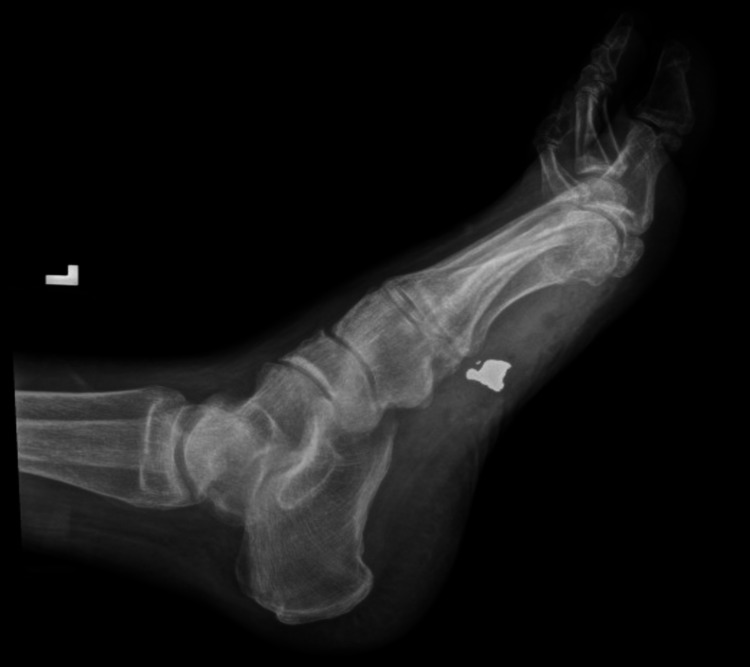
X-ray foot left lateral view (before bullet removal)
